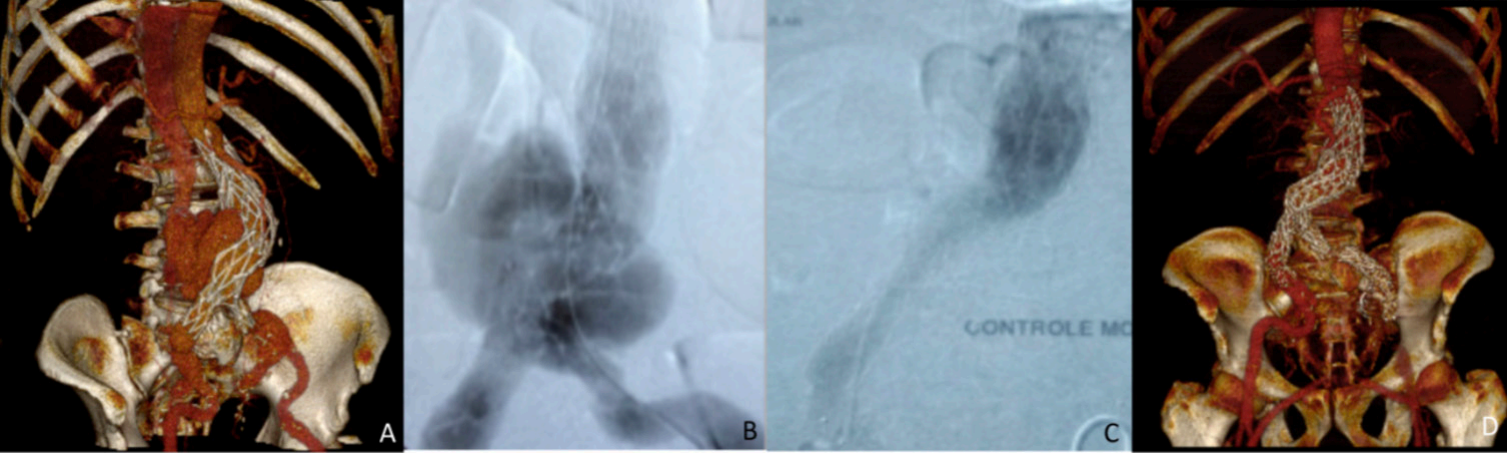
Case #3 (A) Preoperative 3D-CTA showing type 3 endoleak with late rupture related to an AF; (B) Intraoperative DSA showing the type 3 endoleak and AF with filling of the inferior vena cava; (C) Intraoperative DSA demonstrating apparent resolution after endograft deployment; (D) Postoperative 3D-CTA showing complete AF resolution and adequate sealing. AF = Aortocaval fistula; DSA = digital subtraction angiography; 3D-CTA = three-dimensional-by-volume CT angiography.
